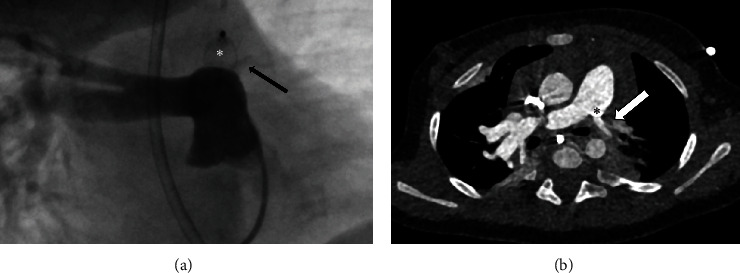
Subtotal obstruction of the left pulmonary artery (arrows) by the ductal AVP-2 (asterisks) 4 months after implantation. (a) Transcatheter angiography. (b) Computed tomography angiography.
